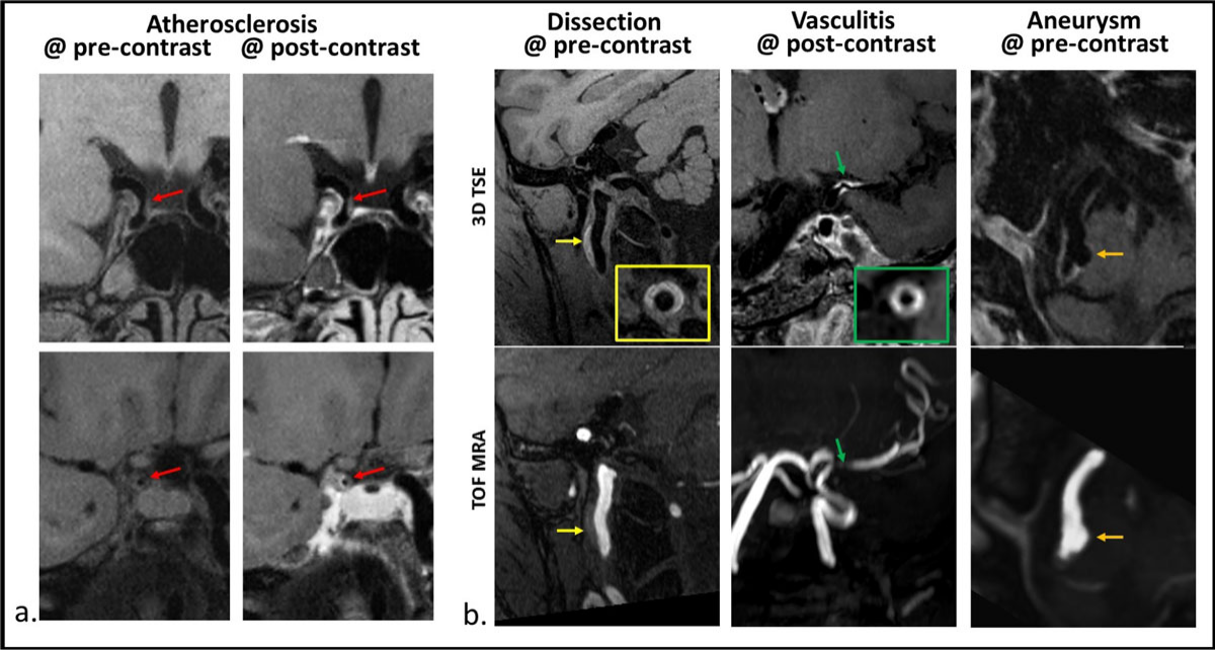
Example clinical images obtained using the optimized whole-brain vessel wall MRI sequence (T1-weighted 3D TSE). The atherosclerotic plaque is depicted by 3D TSE as severe stenosis as well as eccentric wall thickening and contrast enhancement (a). Compared to TOF MRA, 3D vessel wall imaging directly depicts wall pathologies with additional information, such as hyper-intense hematoma associated with dissection and concentric contrast enhancement associated with vasculitis (b). The T1-mediated signal features within various wall pathologies on 3D TSE facilitates definitive diagnosis.
